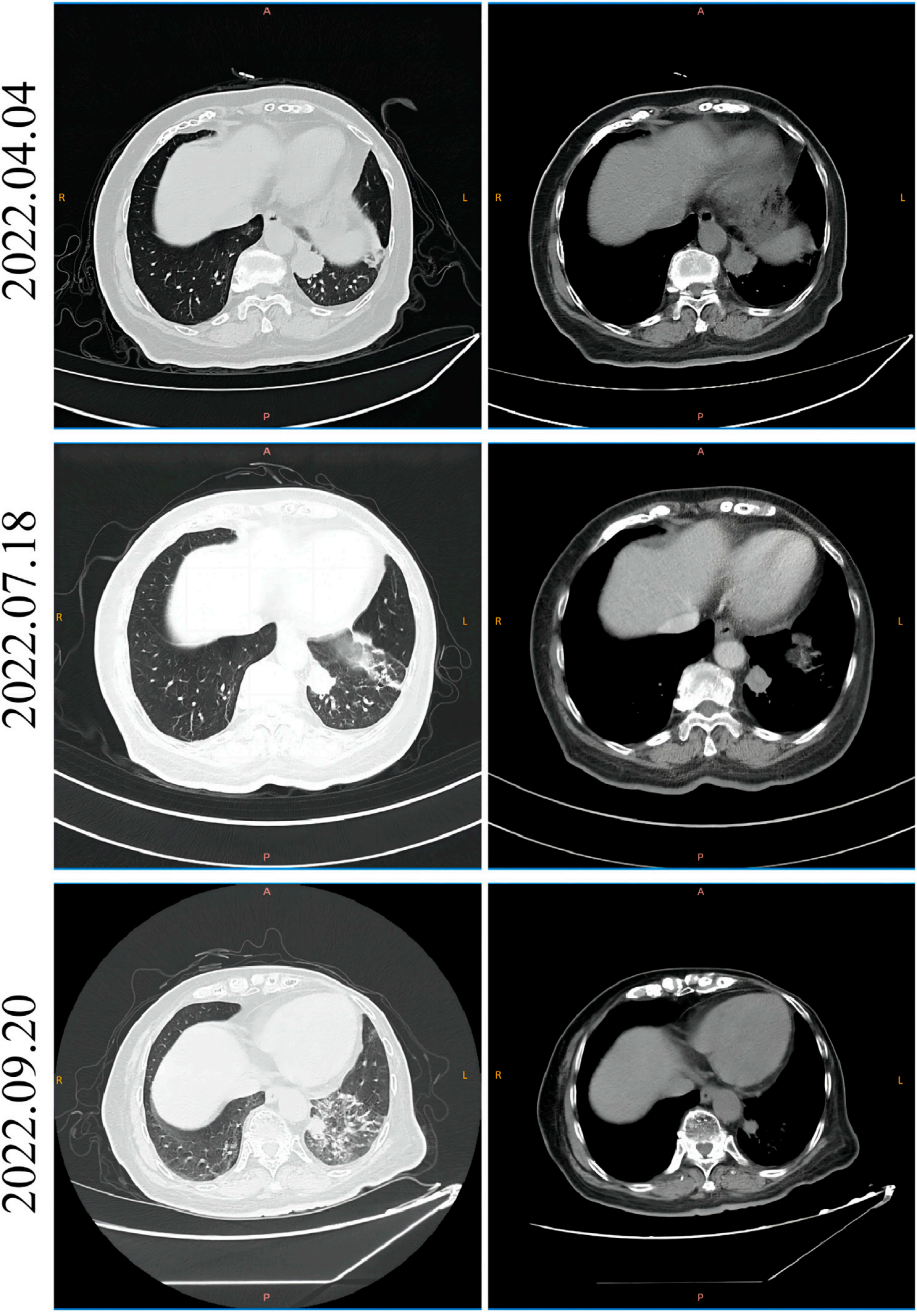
CT imaging of the patient before and after the initial chemotherapy and radiotherapy. The left side is the lung CT scan window, and the right side is the mediastinum CT scan window. The CT image of the patient before receiving chemotherapy on 4 April 2022; the CT image of the patient receiving chemotherapy but not radiotherapy on 18 July 2022; and the CT image of the patient receiving chemotherapy and radiotherapy on 20 September 2022.
